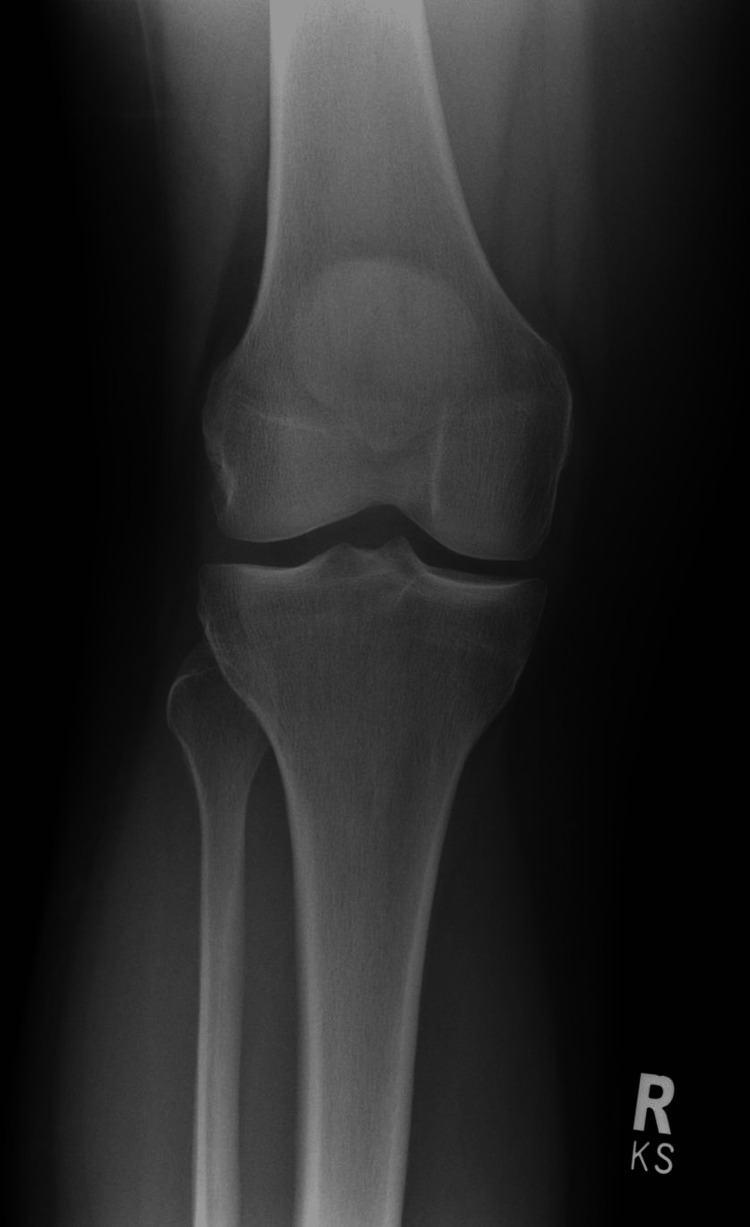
Representative radiograph of Kellgren-Lawrence grade 0 knee osteoarthritis Anteroposterior weight-bearing radiograph showing a normal joint with preserved joint spaces, smooth articular margins, and no osteophyte formation or subchondral sclerosis. This appearance is consistent with Kellgren-Lawrence grade 0, indicating the absence of radiographic osteoarthritis.
